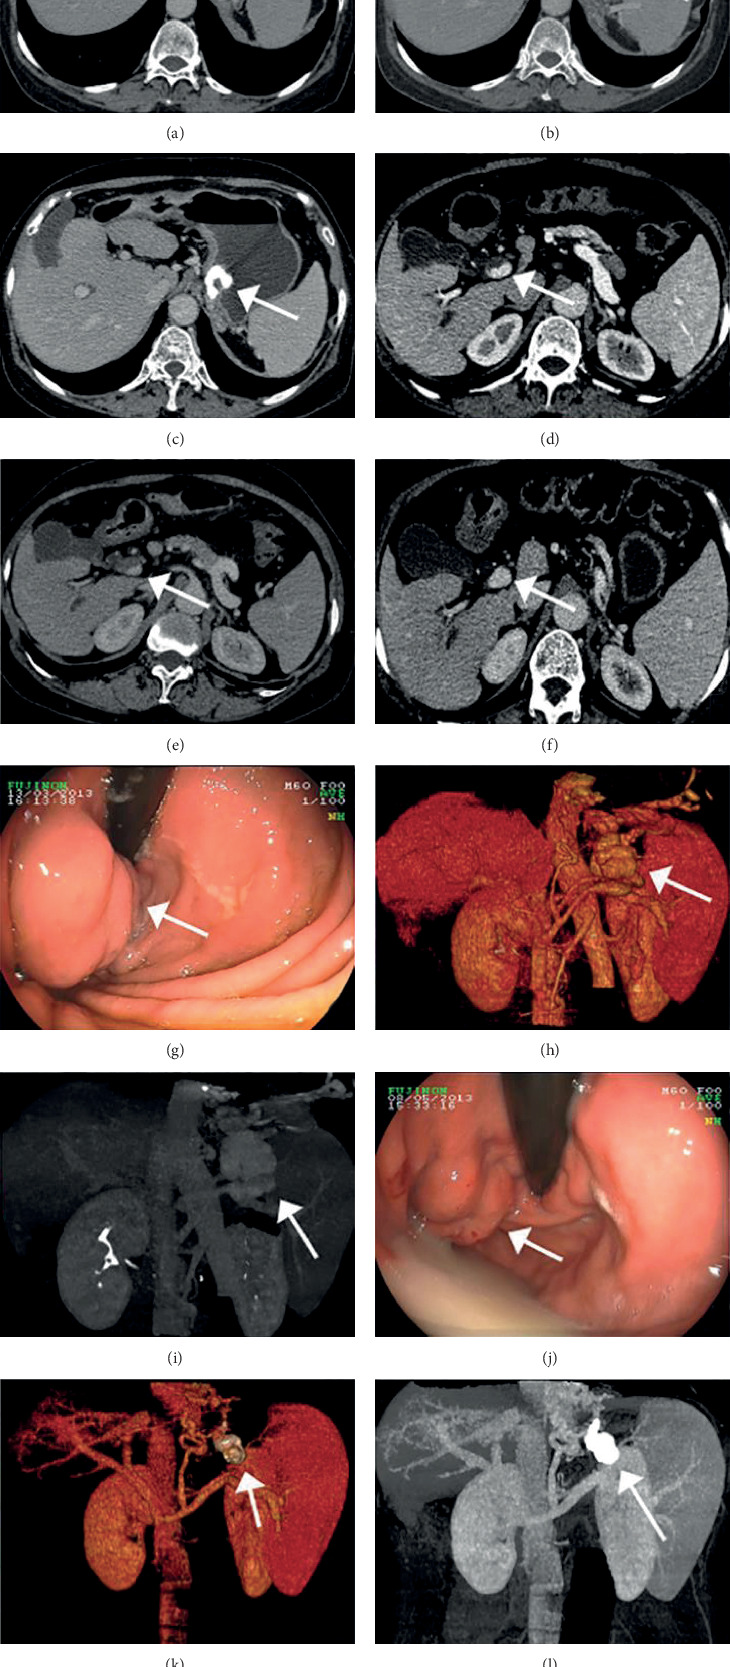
Efficacy of endotherapy in a patient with severe gastric varices. Axial computed tomography images show (a) nodular gastric varices located in the fundus. (b) One week after tissue adhesive injection, the afferent vein is partly embolized (arrowhead). (c) One month later. (d) The patient experienced portal vein thrombosis before treatment. (e) The filling defect of the portal vein was aggravated 1 week after treatment, and (f) it improved 1 month after treatment. (g, j) Endoscopic images show the changes in the varices 1 month after treatment. The reduced volumes of the gastric varices are shown with (h, k) volume rendering and (i, l) maximum intensity projection three-dimensionally.
